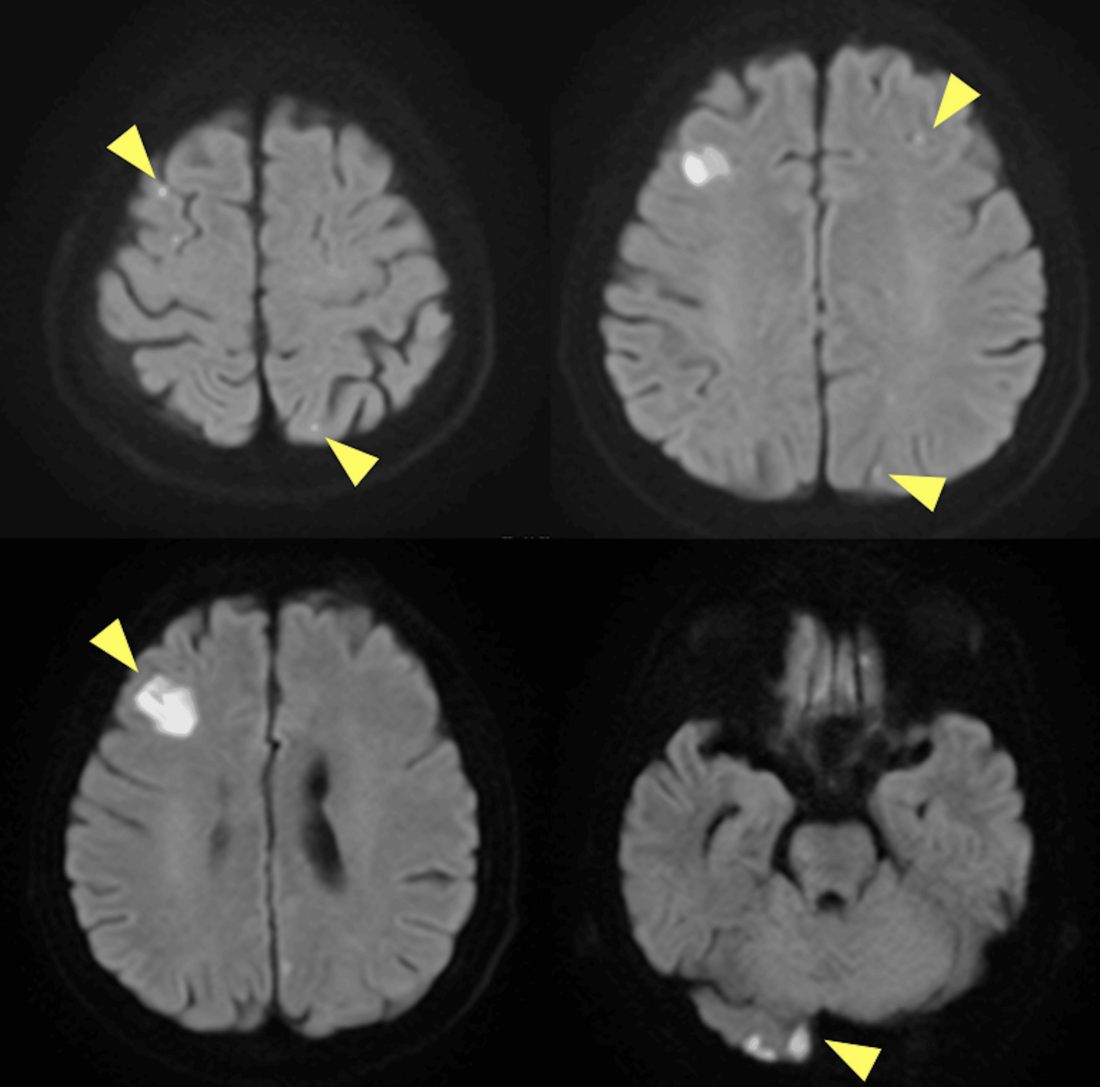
Magnetic resonance imaging of the whole brain There are multiple acute cerebral infarctions throughout the whole brain (yellow arrows).
